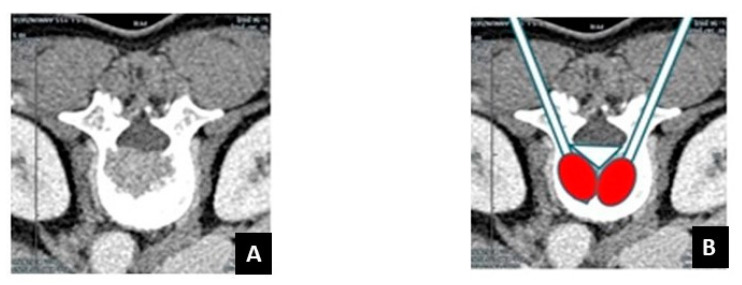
Limits of the use of conventional radiofrequency straight needles for the treatment of difficult-to-reach vertebral metastases. (A) Breast cancer metastasis in a 61-year-old patient. Axial CT scan shows a lytic lesion of in the posterior wall of the vertebral body L2 with cortical disruption. (B) Conventional RFA with straight needles is not able to ablate the entire lesion (white triangle).
